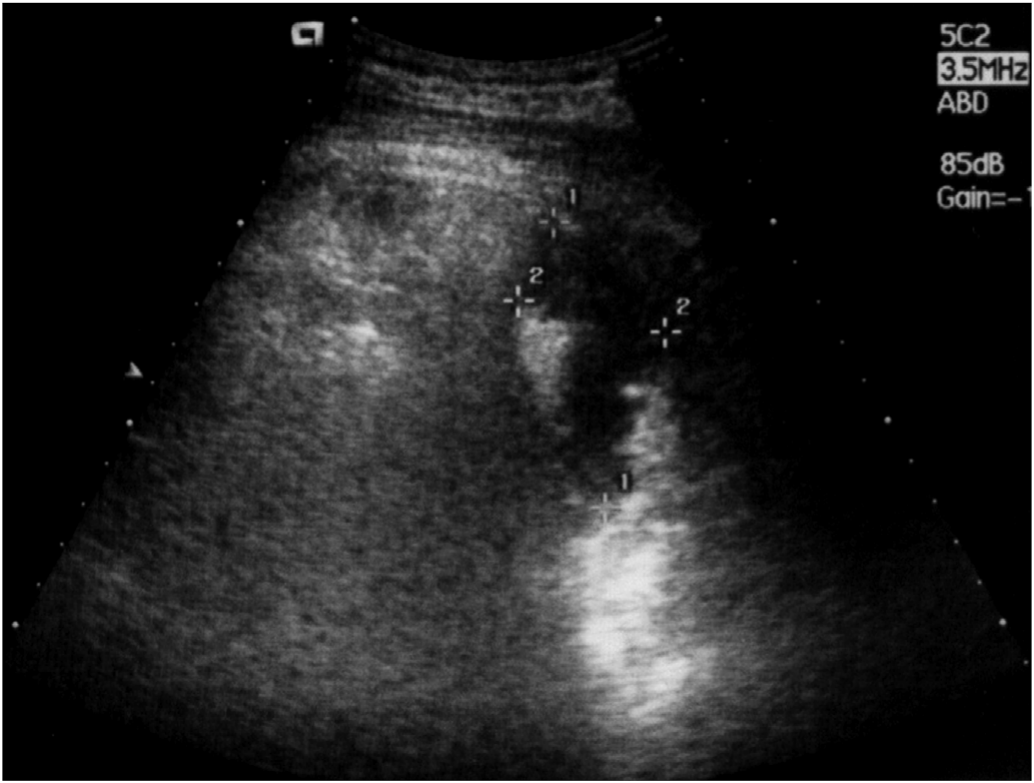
Ultrasound scan of the left-sided subphrenic abscess (indicated by crosses).
